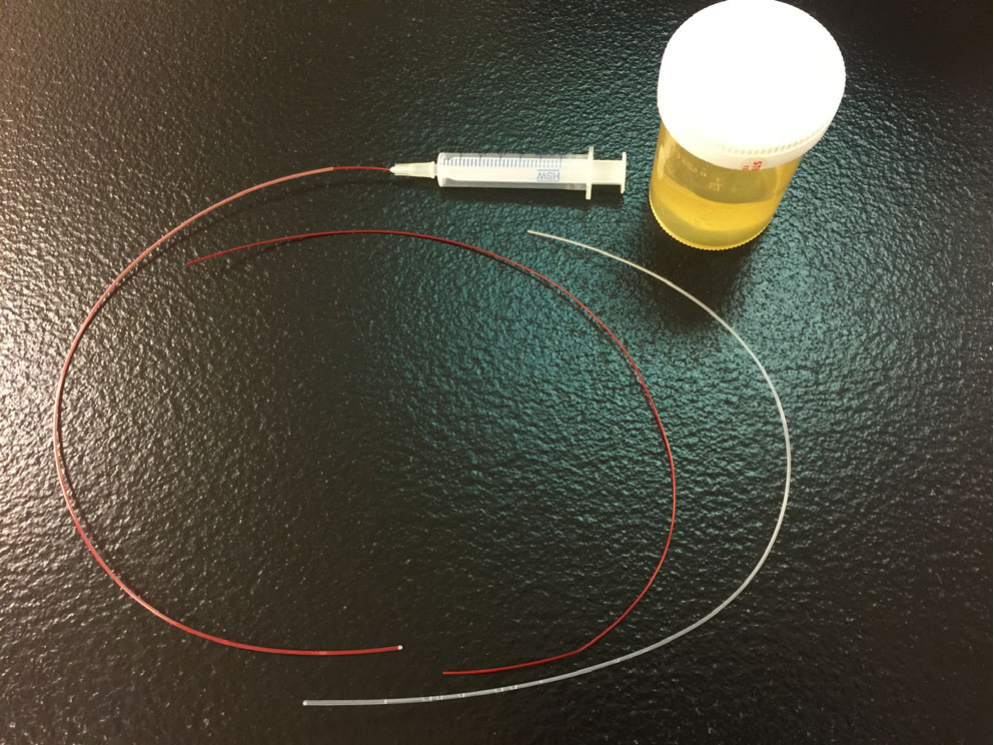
Equipment used to make the line markers of MRI‐HDR‐IBT MRI. Inside the plastic bottle is the prepared T2 contrast agent: medical saline plus several drops of iodine. The larger diameter plastic tubes are the line markers, and the smaller diameter red tubes are the tool used for filling saline into the line markers via the syringe.
